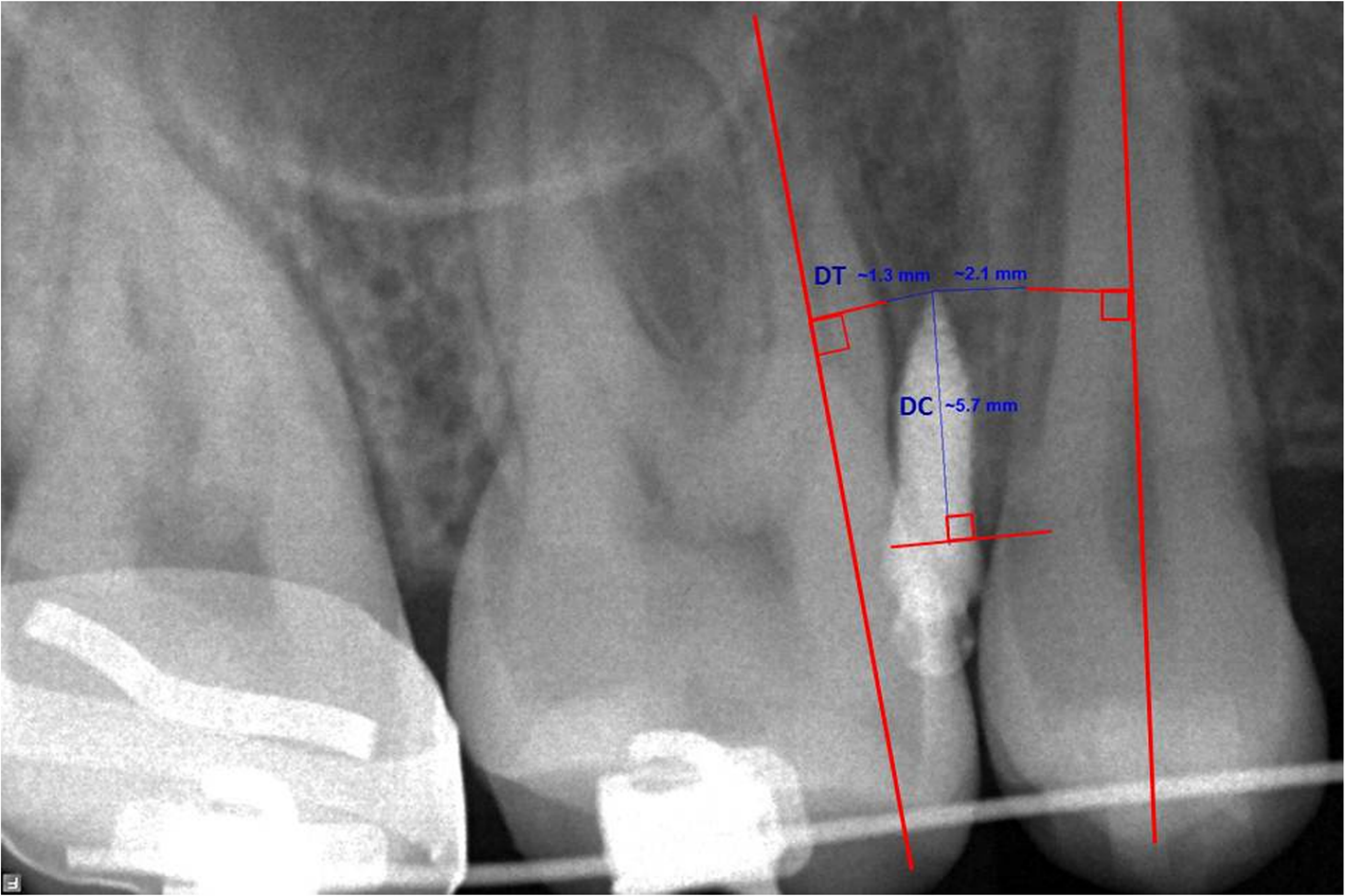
Radiographic evaluation using a periapical radiograph
